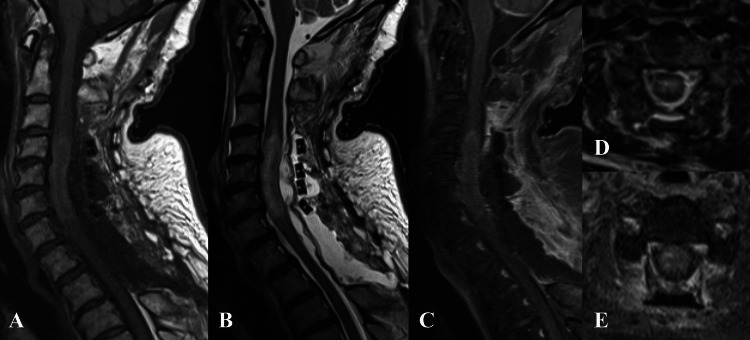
Postoperative gadolinium-enhanced MRI of the cervical spine The dural sac is decently expanded, but the intradural signal change at the C4-Th1 level resides (arrowhead). Aː Sagittal T1 fat-saturated weighted image; Bː Sagittal T2-weighted image; Cː Sagittal gadolinium-enhanced image; D: Axial T2-weighted image at the C4/5 level; E: Axial gadolinium-enhanced image at the C4/5 level
